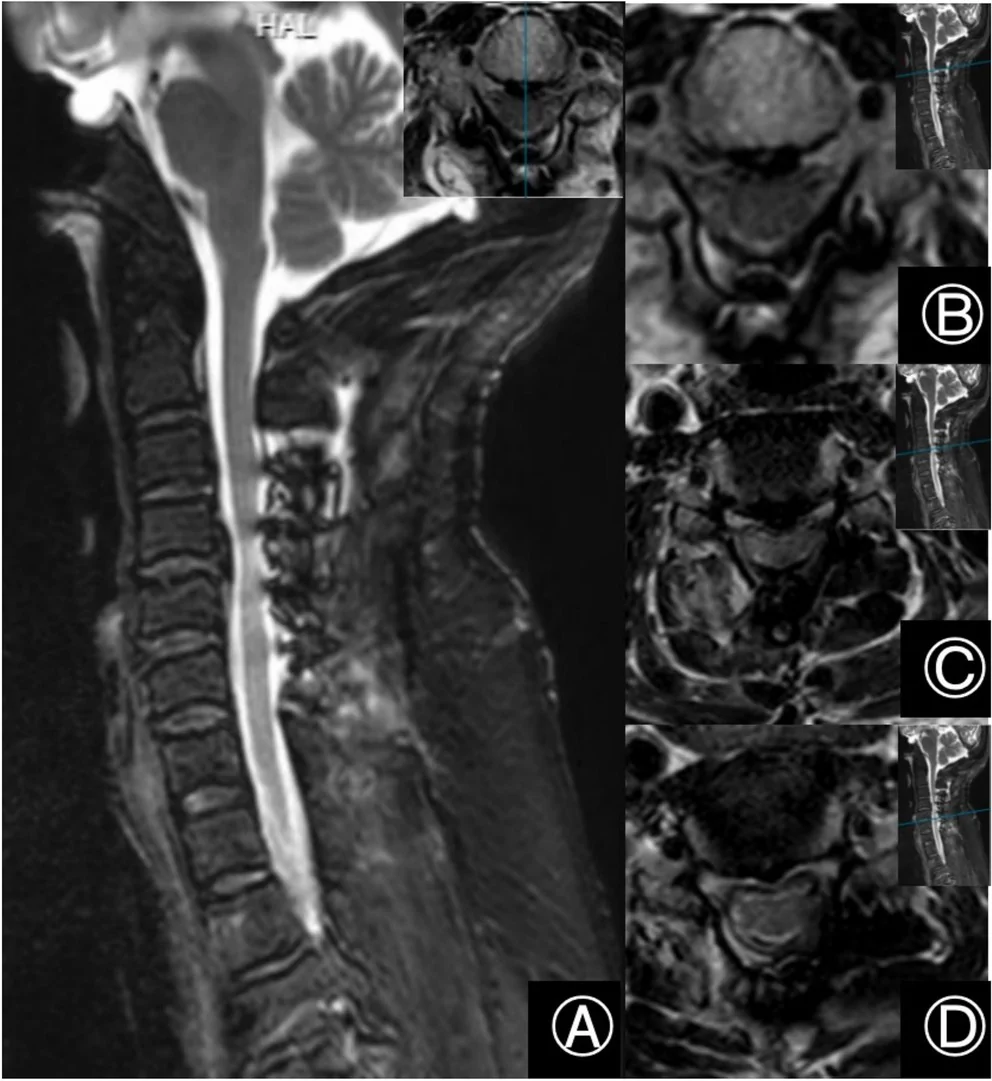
The follow‐up MRI on postoperative Day 3 revealed spinal canal expansion with posterior migration of the spinal cord, absence of cord compression, and no significant hematoma posterior to the vertebral bodies or within the spinal canal.
